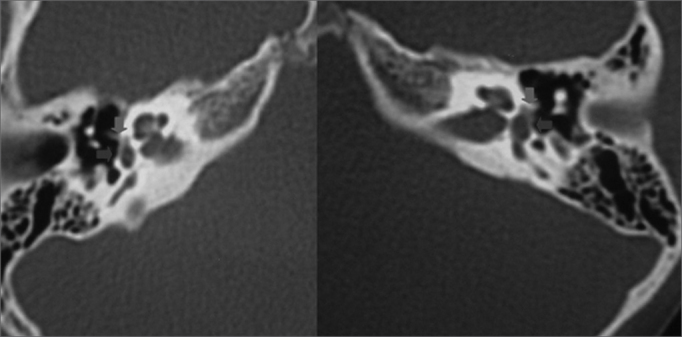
CT of the temporal bones in axial sections showing a small hypodensity in the area anterior to the oval window (green arrows) and thickening of the stapes footplate (blue arrows).
